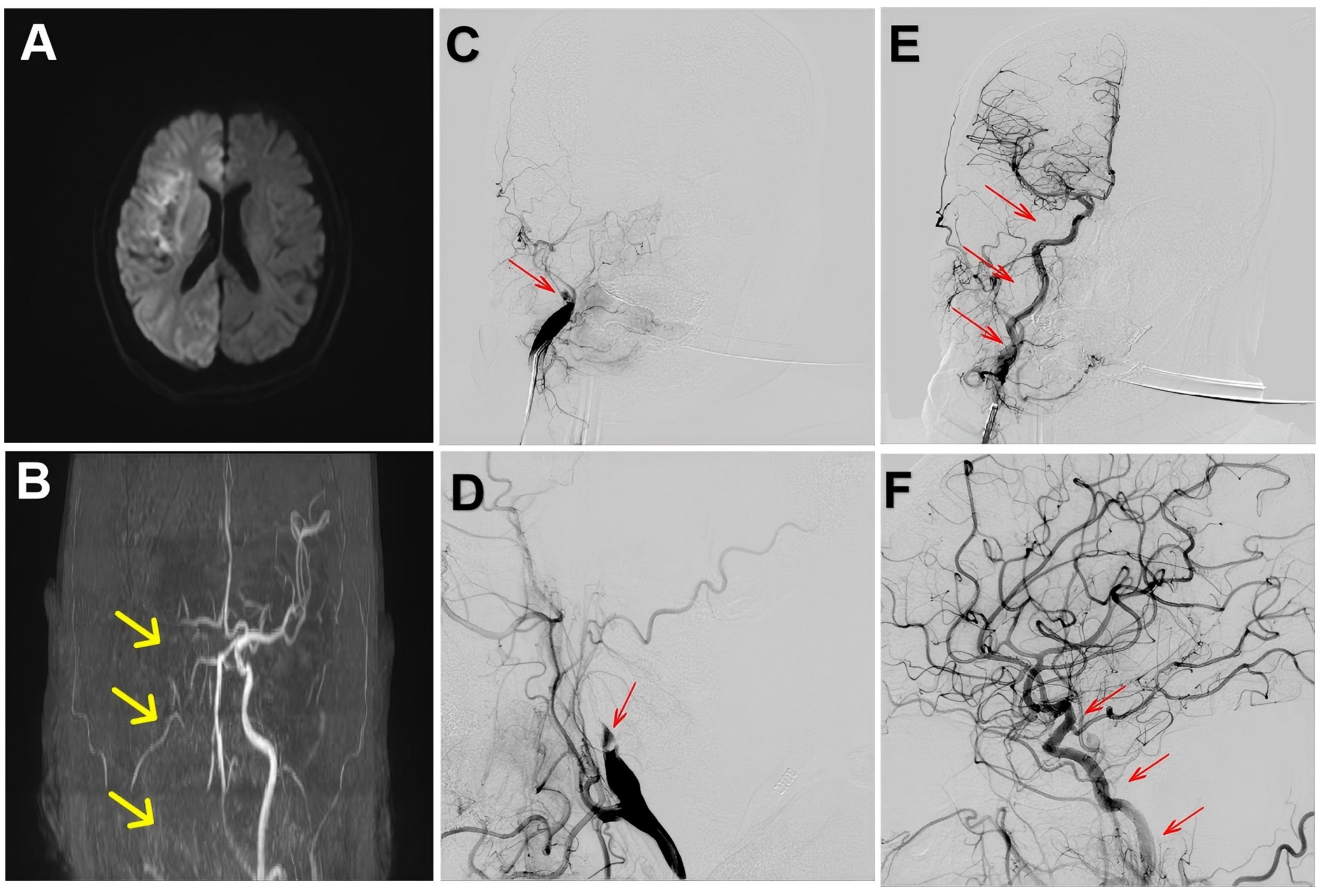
(A) Brain MRI showing a large infarction in the right cerebral hemisphere. (B) Brain MRA demonstrating occlusion of the right internal carotid artery, indicated by the yellow arrow. (C,D) Right cerebral angiography showing occlusion of the right internal carotid artery, indicated by the red arrow. (E,F) Right cerebral angiography showing restoration of blood flow in the right internal carotid artery, indicated by the red arrow.
